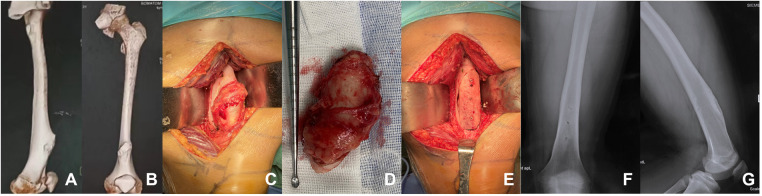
A patient with femoral osteochondroma. (A,B) The preoperative three-dimensional reconstruction of the patient showed a huge osteochondroma at the left femoral. (C–E) Osteochondroma resection was performed from the base of the tumor. The allograft was implanted in the bone cortical defect and fixed with three absorbable screws. (F,G) One year after the operation, the radiographs of the left femur showed that the effect of bone graft fusion was satisfactory.
